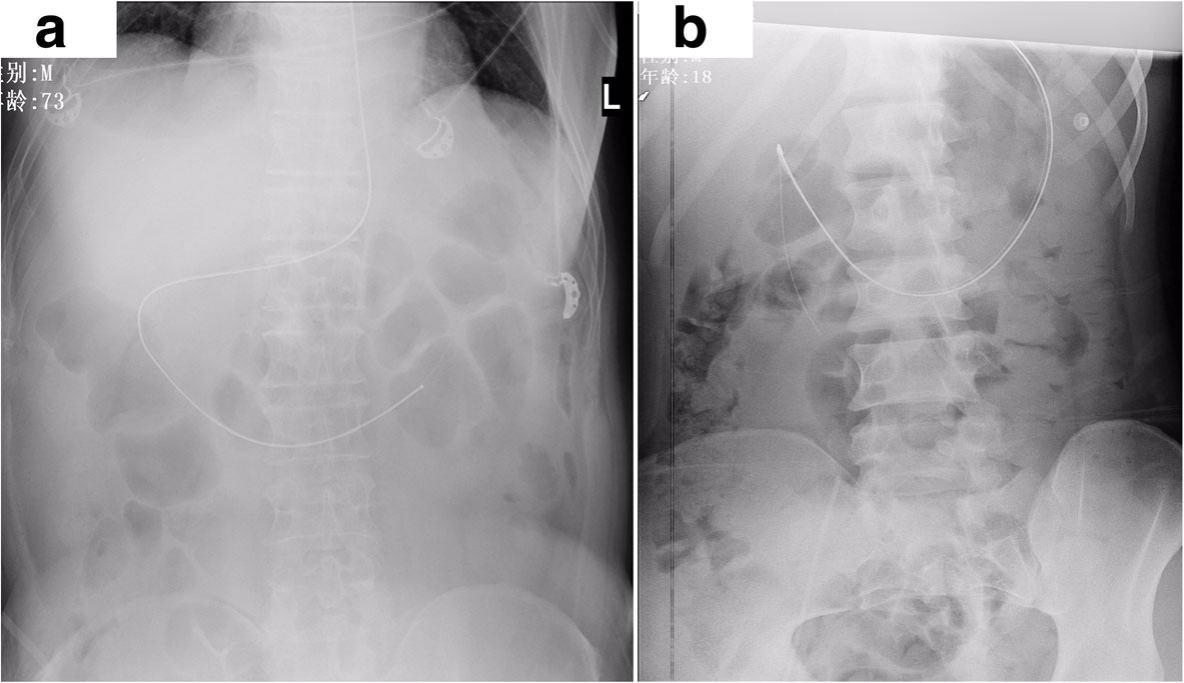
Abdominal plain radiographs showing the tip of the Flocare tube positioned at the horizontal part (a) and descending part (b) of the duodenum. The former demonstrates the classic C-shaped duodenal configuration diagnostic of postpyloric placement
